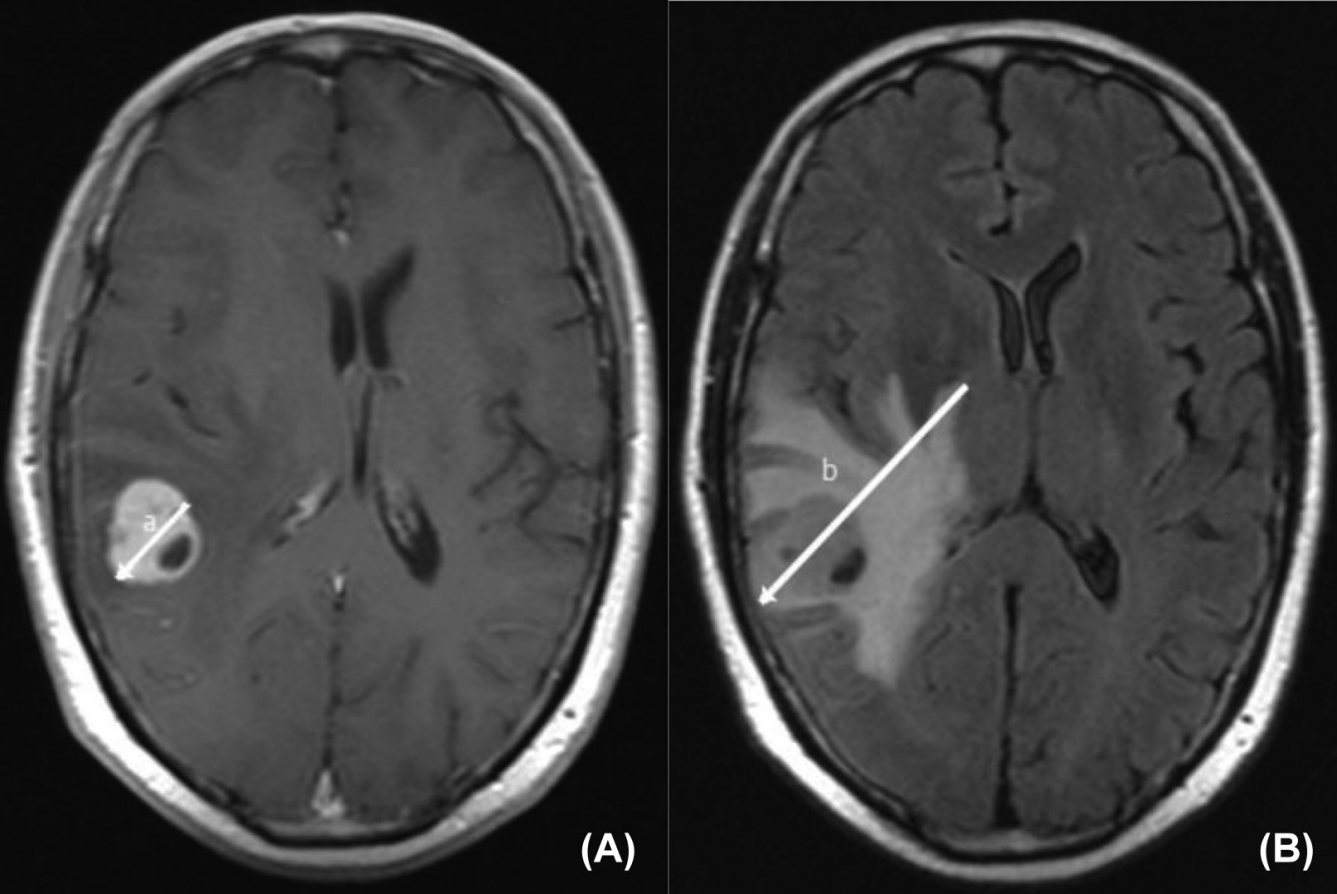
Method of measuring brain metastasis. (A) The maximum diameter of the tumor was measured via T1‐weighted Gd‐enhanced imaging (unidirectional arrow labeled “a”); (B) Peritumoral brain edema size was measured via T2‐FLAIR (unidirectional arrow labeled “b”).
